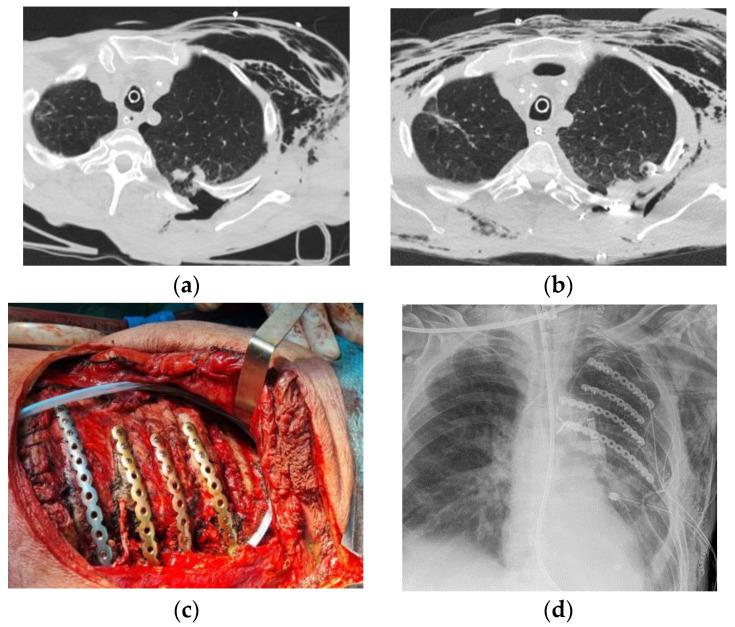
(a) Preoperative computed tomography disclosing rib fracture and penetrating lung injury. (b) Postoperative computed tomography disclosing rib stabilization by plate and screws. (c) Intraoperative view disclosing plates, screws and stitches. (d) Postoperative chest X rays.
